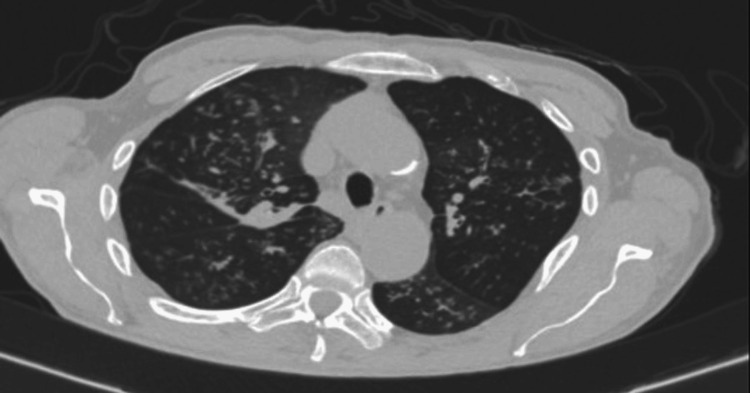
Chest CT showing bilateral micronodules and a tree-in-bud pattern
